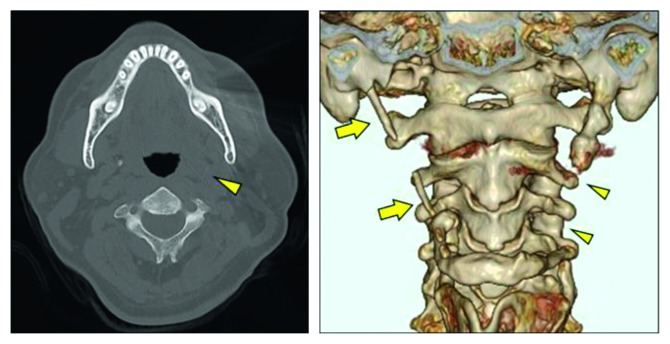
Postoperative computed tomographic image and three-dimensional reconstruction. A good portion of the left styloid process was resected (arrowheads). The styloid process on the right side was also elongated, and the stylohyoid ligament was partially calcified (arrows). Ongoing observation is required.
